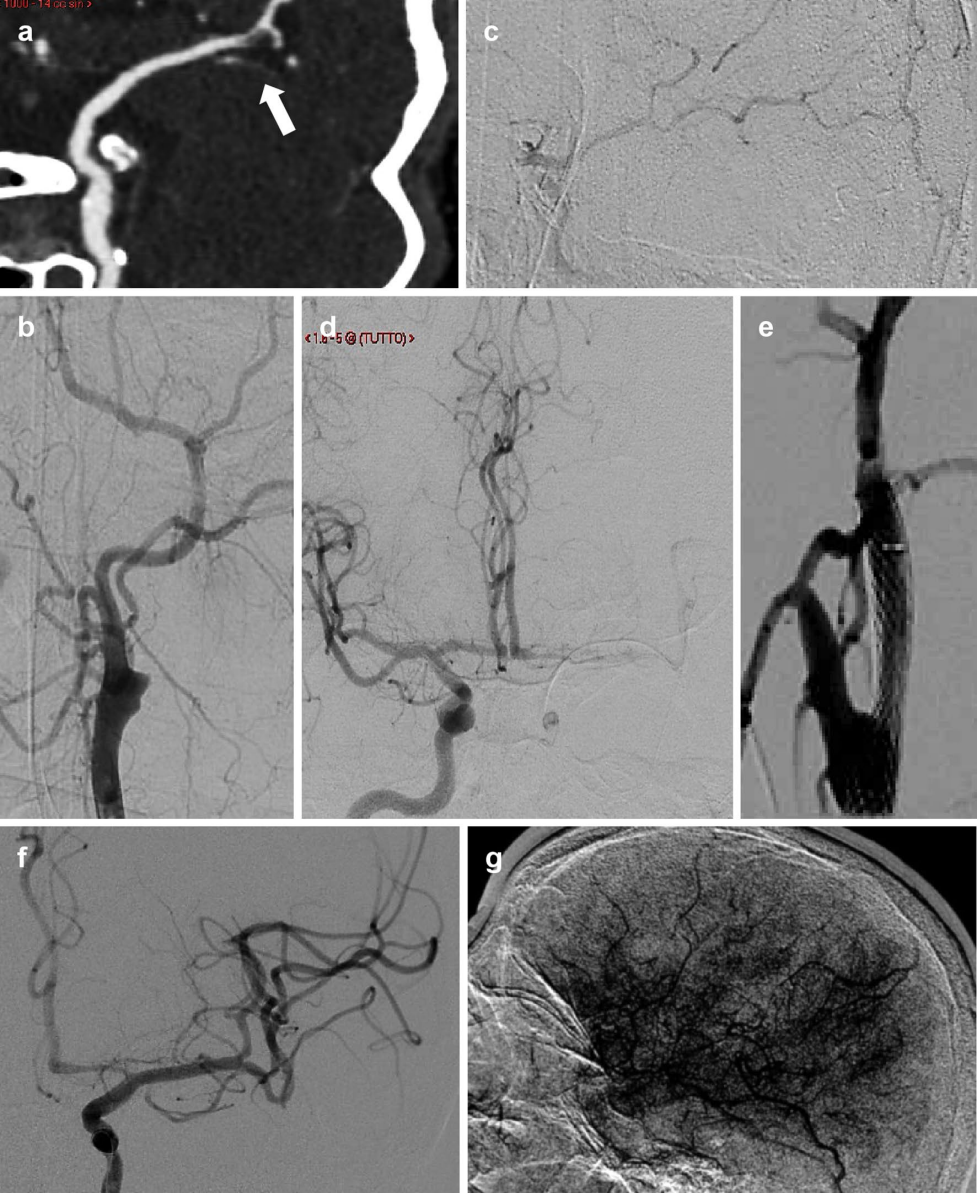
Patient with aphasia, right upper limb hyposthenia and paresthesia (NIHSS 9). Computed tomography angiography, performed at the Spoke Hospital, revealed left M1-MCA sub-occlusion (a, arrow). Patient was centralized for thrombectomy to the Hub Hospital immediately after administration of IV-FL. Digital subtraction angiography revealed a complete occlusion of the left extracranial ICA (b), with normal opacification of the previously sub-occluded vessel via retrograde anastomotic filling from the ipsilateral ophthalmic artery (c). For these reasons, intracranial thrombectomy was not carried out. Since hemodynamic impairment was observed, as poor activation of Willis’ circle (d), it was decided to perform eCAS (e). Post-procedure angiography showed good cerebral reperfusion (f, g), mTICI 2b. 90-day mRS 0
